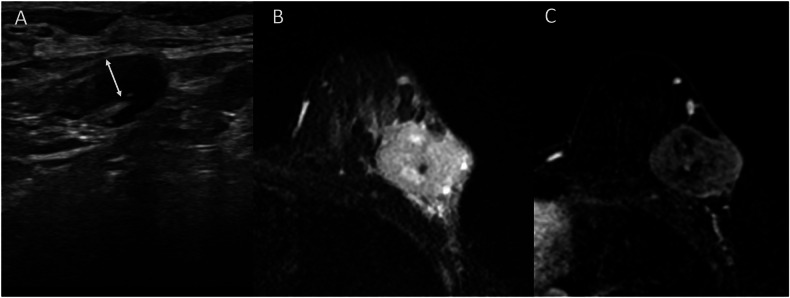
Triple-negative breast cancer in a 44-year-old patient, with a KI-67 equal to 90%. Baseline axillary ultrasound shows an adenopathy with a 4 mm cortical thickening (A). On MRI examination, the tumor in the posterior part of the breast includes high central signal intensity areas. It is surrounded by peritumoral edema on T2-weighted images (B), with a heterogeneous enhancement without non-mass enhancement on subtracted images from dynamic contrast-enhanced T1-weighted acquisitions (C). The patient achieved an axillary complete response after neoadjuvant chemotherapy, RCB0. RCB, residual cancer burden
